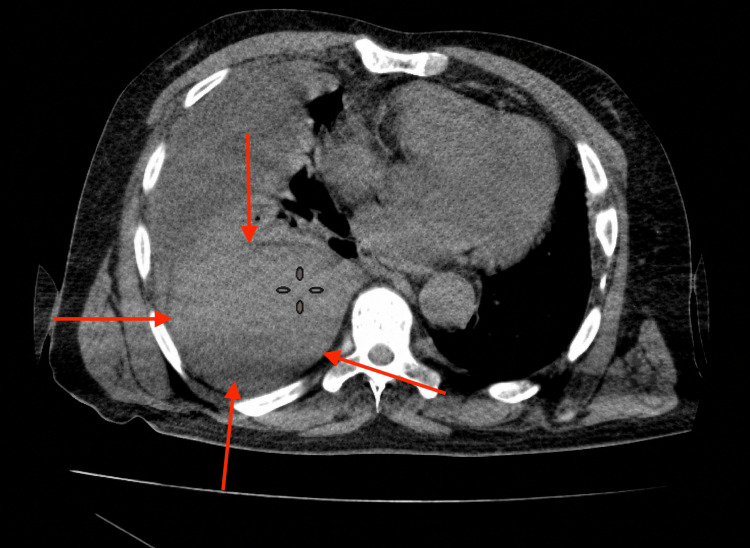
Coronal non-contrast CT image showing a right-sided peripheral extrapleural collection with lenticular morphology. The arrow indicates the extrapleural hematoma, and the downward arrow highlights displaced extrapleural fat, corresponding to the extrapleural fat sign.
